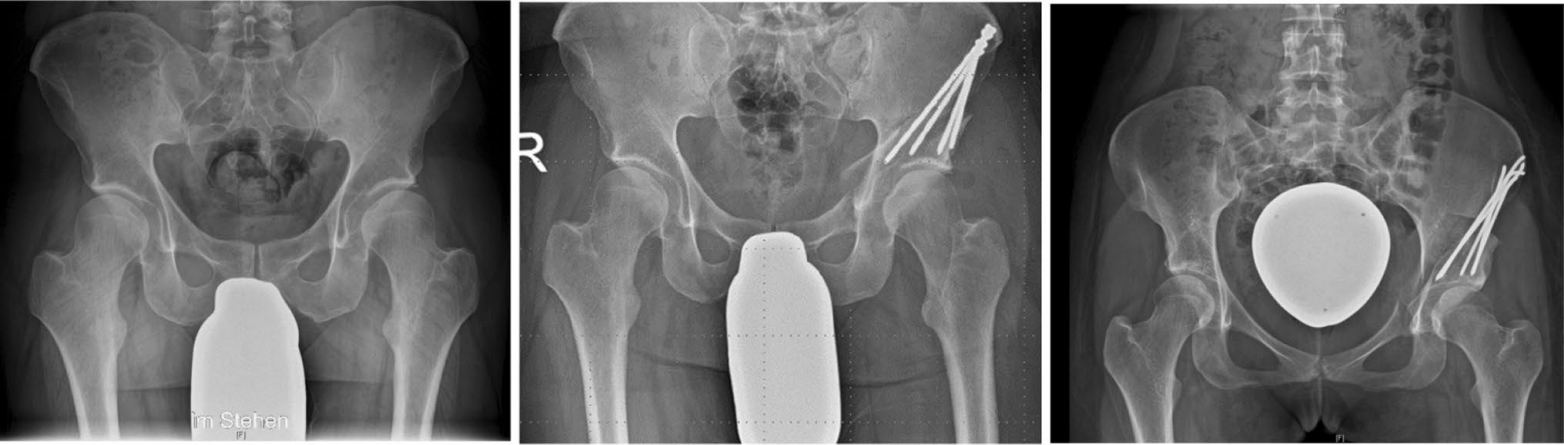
a Preoperative radiographic assessment for indication of PAO. b Correction and fixation with screws. c Correction and fixation with K-wires
